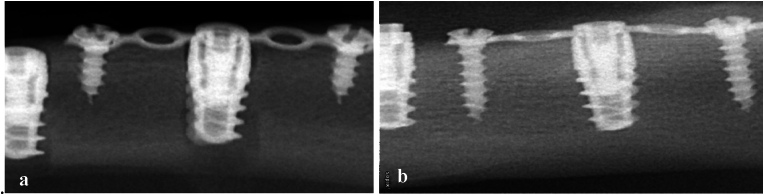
Digital x-ray images (scale bar 10 mm) showing dental implants on day of insertion. Notice larger osteotomy site appearing around compromised implant on the right (a). Digital x-ray image showing the compromised dental implant after 8 weeks. Notice complete healing of the large osteotomy site (b).
